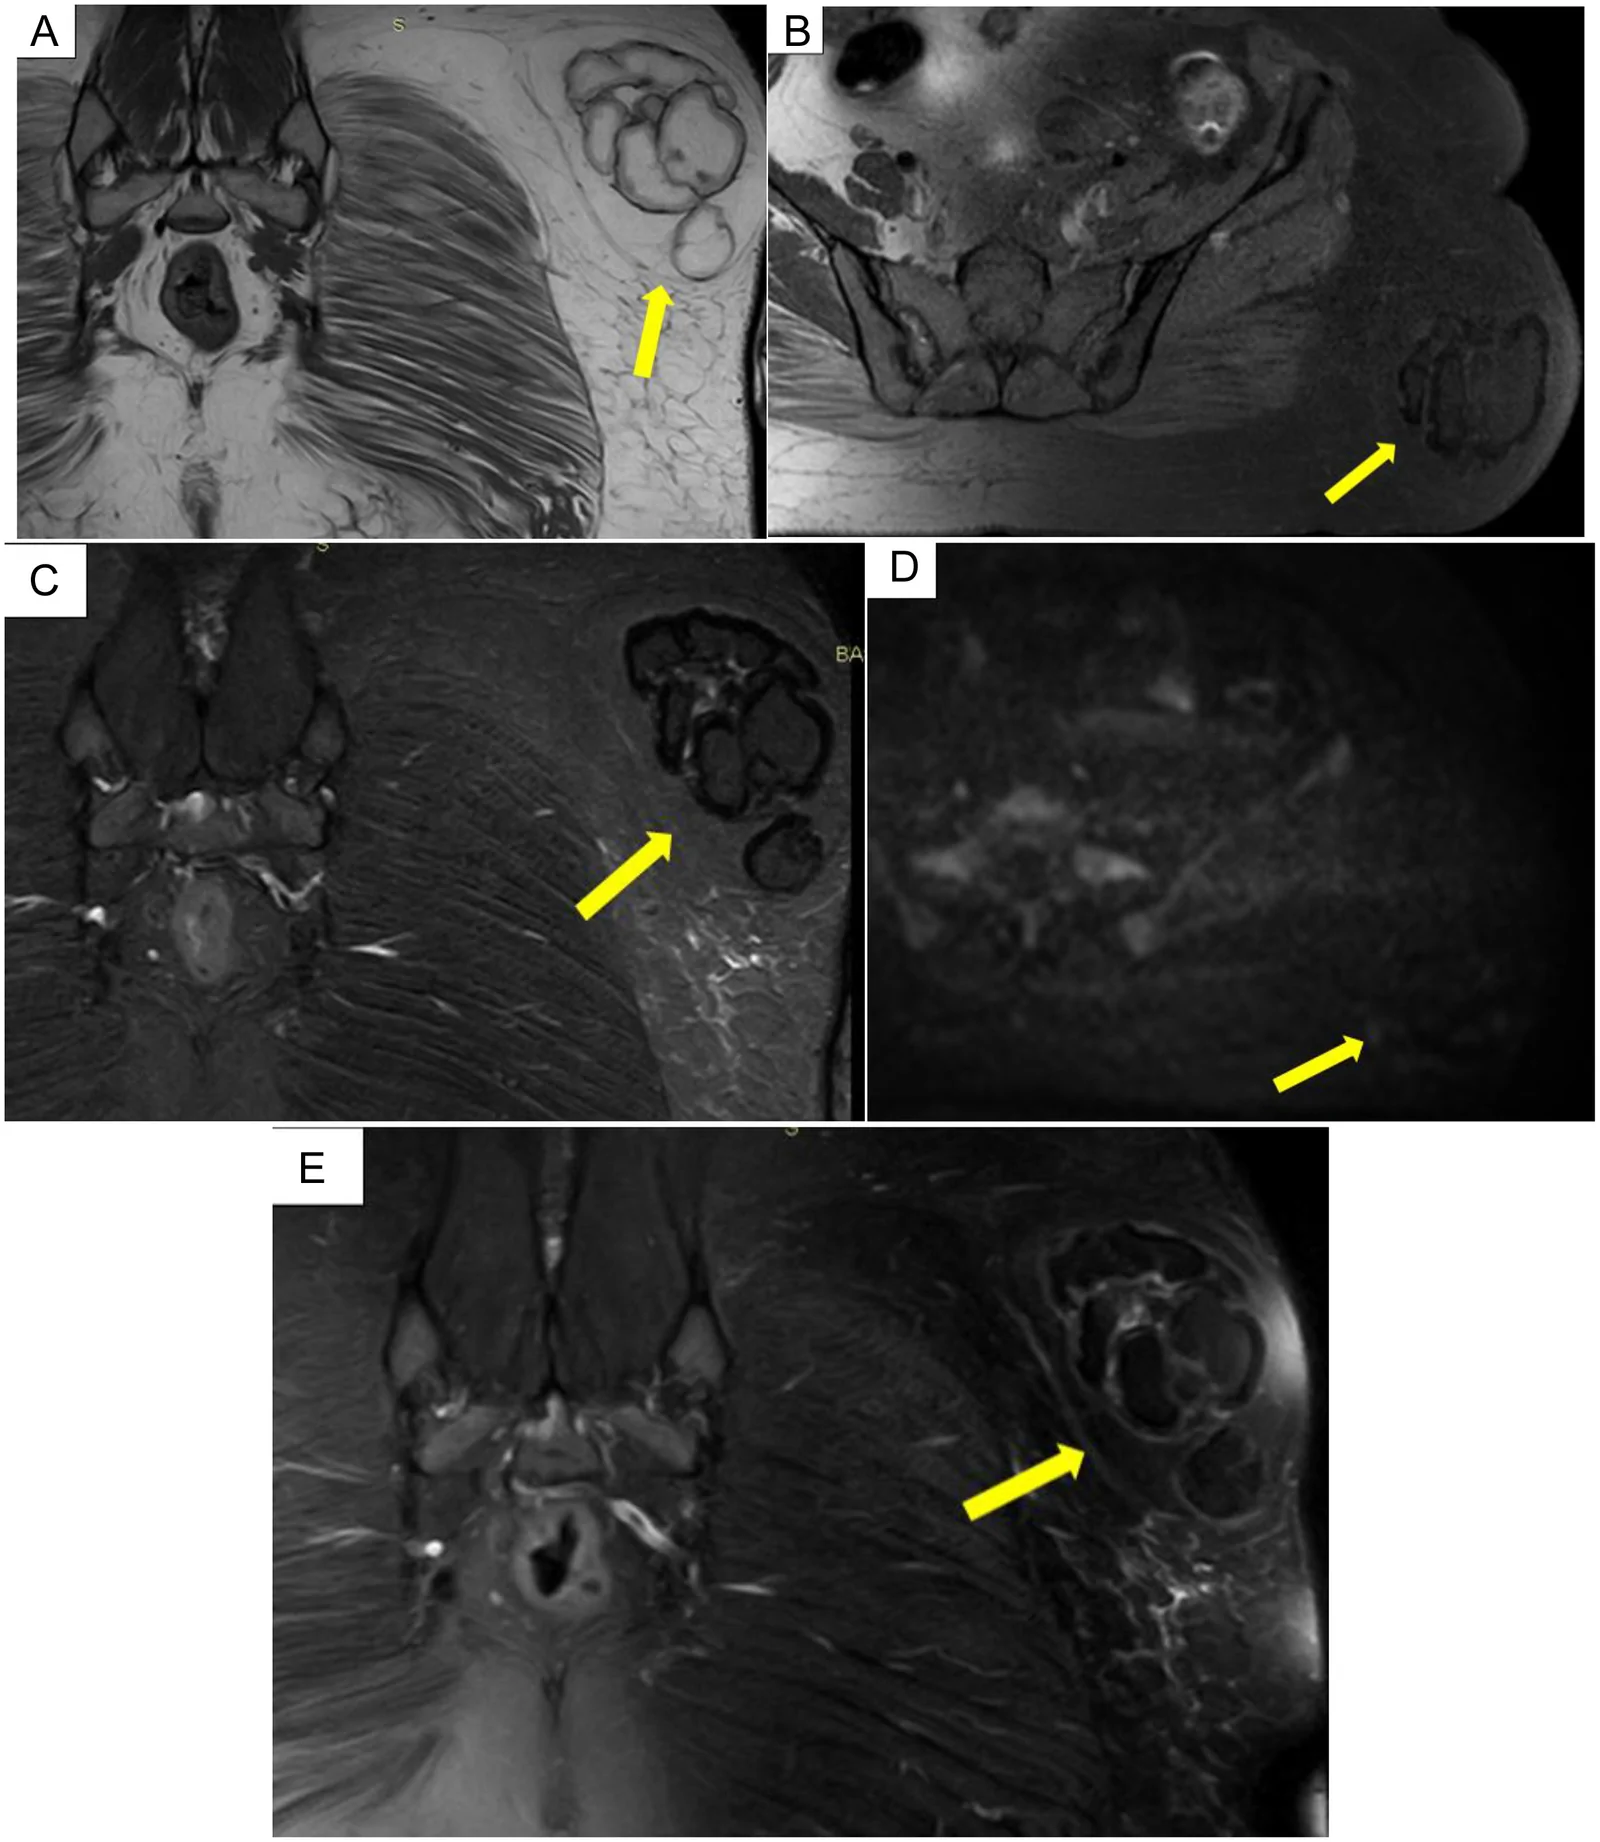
Pelvic MRI including coronal T1-weighted (A), axial T1 fat-saturated (B), coronal STIR (C), axial diffusion-weighted imaging (D), and contrast-enhanced coronal T1 fat-saturated (E) images, demonstrating a well-defined lobulated lesion confined to the subcutaneous tissue of the superolateral quadrant of the left gluteal region. The lesion is surrounded by a thin hypointense capsule visible on all sequences and contains a central fatty component that is hyperintense on T1-weighted images, with complete signal suppression on fat-saturated sequences, including STIR. No internal enhancement is observed after gadolinium administration (yellow arrow).
